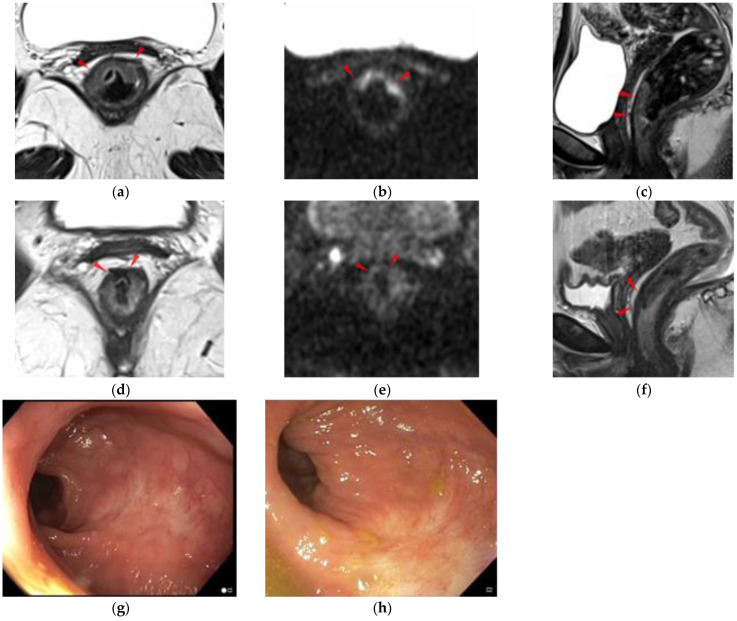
A 40-year-old female patient, initially staged mrLR3CRM+N+N1c+EMVI-, with a complete response at restaging MRI and endoscopy. (a–c) Staging MRI: axial T2WI HR (a), axial DWI (b), sagittal T2WI (c) showing a low rectal tumor (red arrowheads); (d–f) Restaging MRI after nCRT: axial (d) and sagittal T2WI HR (f) showing a pronounced reduction in size with positive split scar sign (SSS+, red arrowhead), score 0 on T2WI. No residual hyperintense signal on b ≥ 1000, with score 0 on DWI (e); (g,h) Flat white scar with telangiectasias at endoscopy, in accordance with clinical complete response.
